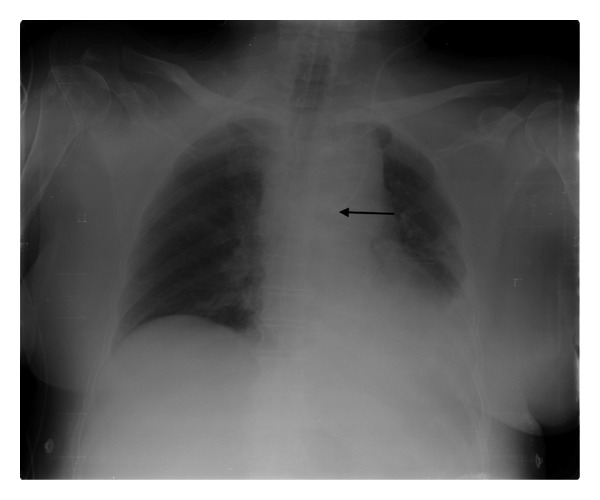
Chest radiograph showing tip (arrow) projecting over the left side of the descending aorta, at the level of carina, creating an angle with the spine greater than 40°.
